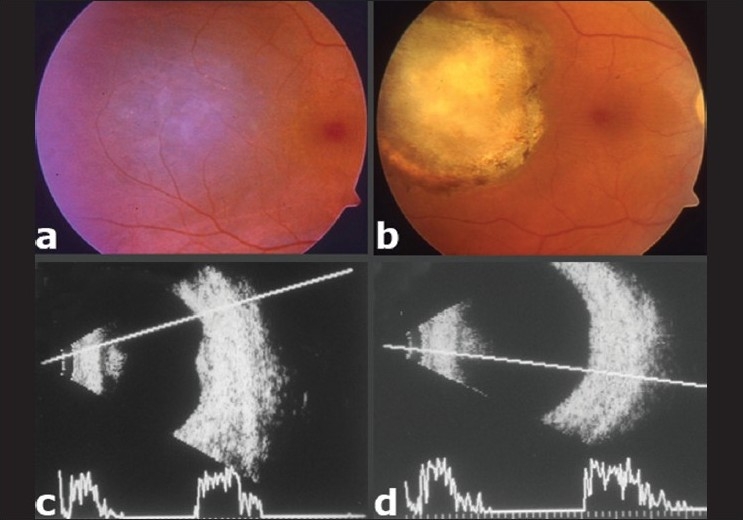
(a) Pigmented choroidal melanoma located temporal to the fovea. (b) Complete regression of the melanoma into a flat chorioretinal scar after three sessions of transpupillary thermotherapy. (c) B-mode ultrasonogram shows a 2.5 mm-thick choroidal melanoma with acoustic solidity at baseline. The vitreous appears clear and there is no extraocular extension. (d) B-mode ultrasonogram after three sessions of transpupillary thermotherapy demonstrates complete regression of choroidal melanoma
